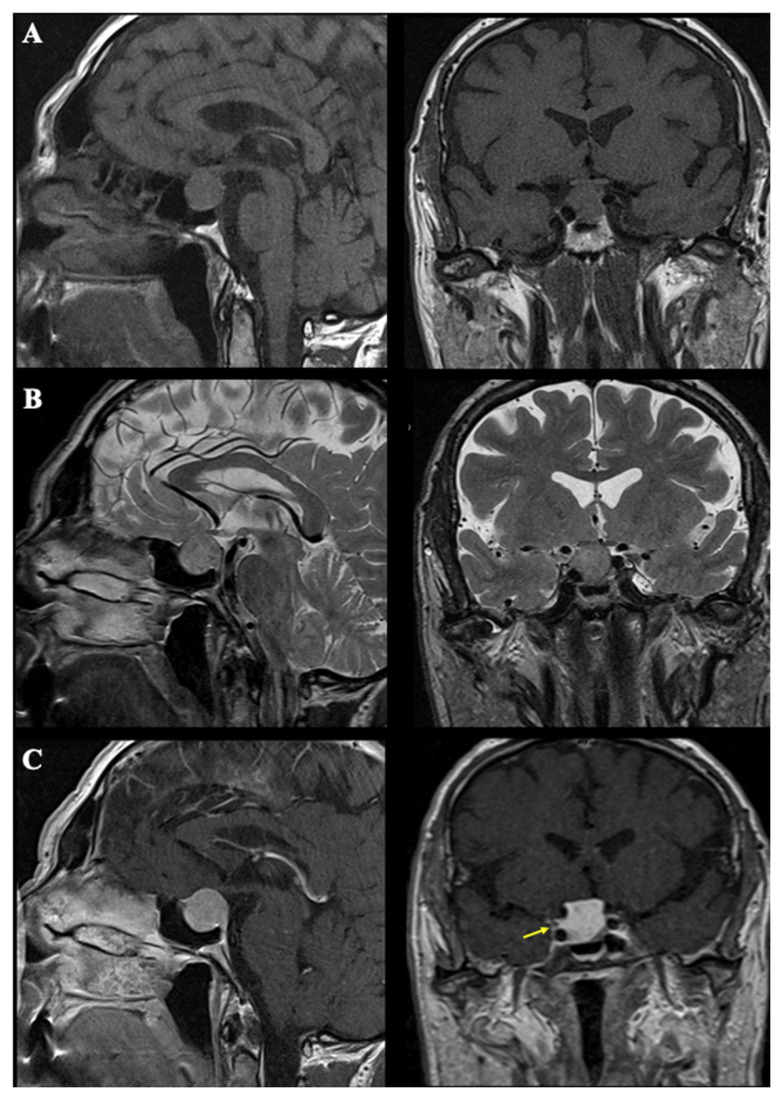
Preoperative magnetic resonance imaging of the sellar solitary fibrous tumor. (A) Sagittal (left) and coronal (right) T1-weighted images demonstrate an iso- to hypointense sellar mass with suprasellar extension. (B) Sagittal (left) and coronal (right) T2-weighted images show predominantly isointense signal intensity. (C) Contrast-enhanced sagittal (left) and coronal (right) T1-weighted images demonstrate heterogeneous enhancement with parasellar extension and right cavernous sinus invasion (Knosp grade II, yellow arrow).
